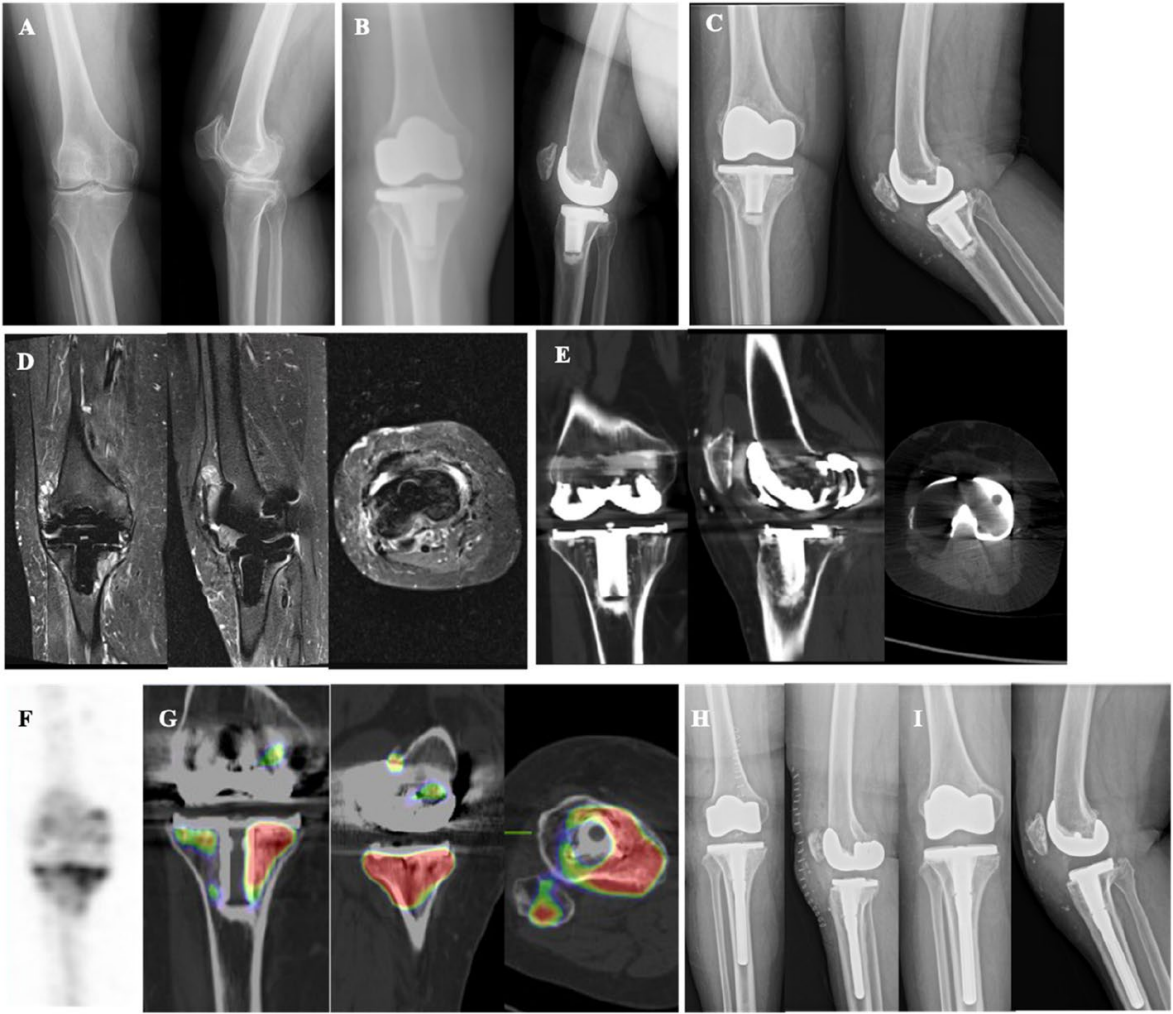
A revision surgery case. A Standing anteroposterior and lateral radiographs of the right knee demonstrate narrowing of the joint space, consistent with osteoarthritic (OA) changes. B Post-TKA anteroposterior and lateral radiographs of the right knee show a prosthesis in satisfactory position with normal alignment. C Anteroposterior and lateral radiographs of the right knee 15 years after the primary TKA. D CT scan of the right knee 15 years post-TKA. E MRI of the right knee 15 years post-TKA. F Triple-phase bone scan reveals radioactive concentration in the right knee joint. G SPECT/CT imaging identifies focal radionuclide concentration in the lateral tibia. H Intraoperative exploration discloses impingement between the lateral articular surface of the left patella and the lateral condyle of the femoral prosthesis, with cartilage defects and metal debris attachment. I Standing anteroposterior and lateral radiographs following revision surgery. J Standing anteroposterior and lateral radiographs at a one-year follow-up post-revision
